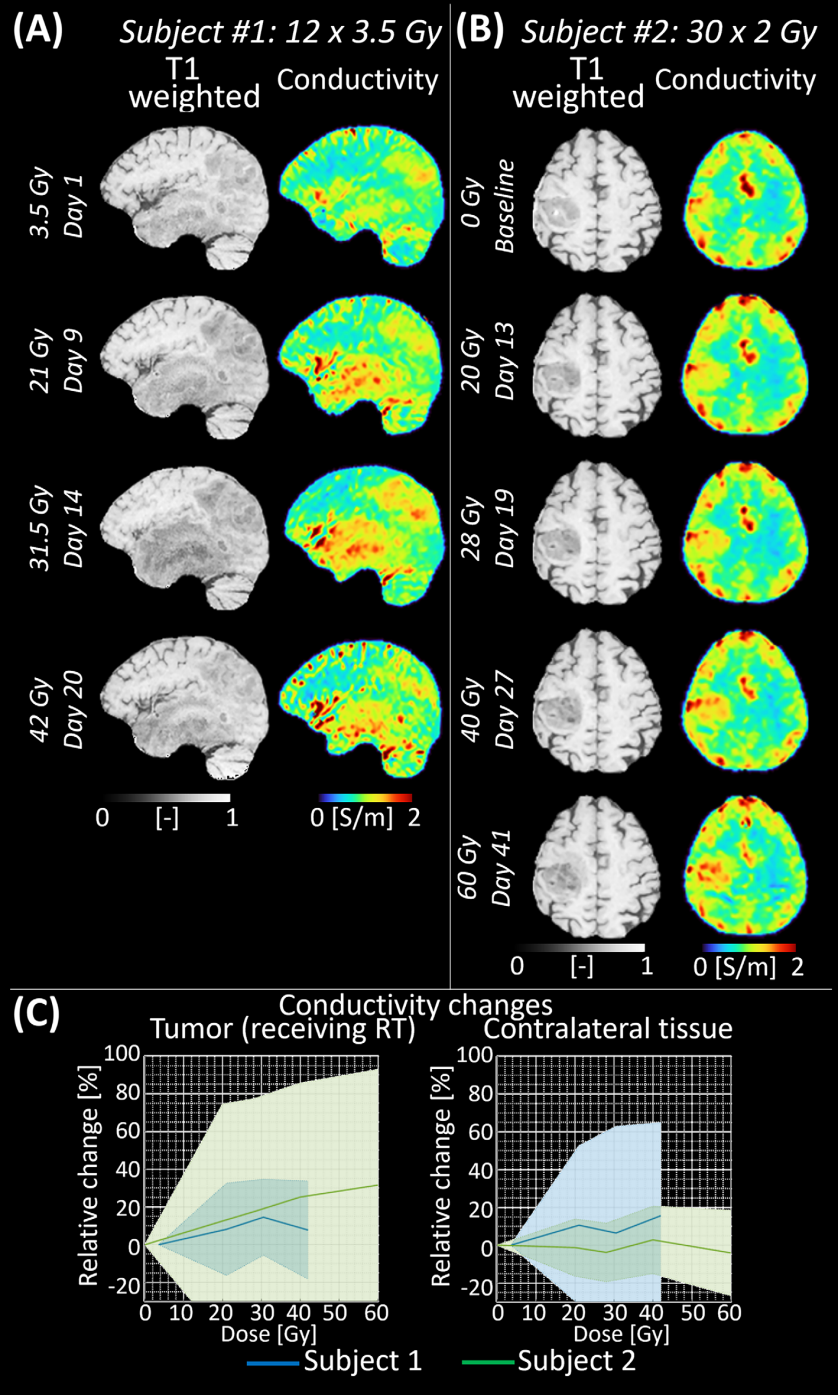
Example of conductivity changes during radiotherapy for two glioblastoma grade IV, IDH‐wildtype. (A, B) Qualitative images are shown for each MRI during the weeks of radiotherapy (RT). (C) Relative percentage difference within the tumor and contralateral tissue, obtained by mirroring the tumor mask and used as a reference for each time point with respect to baseline.
